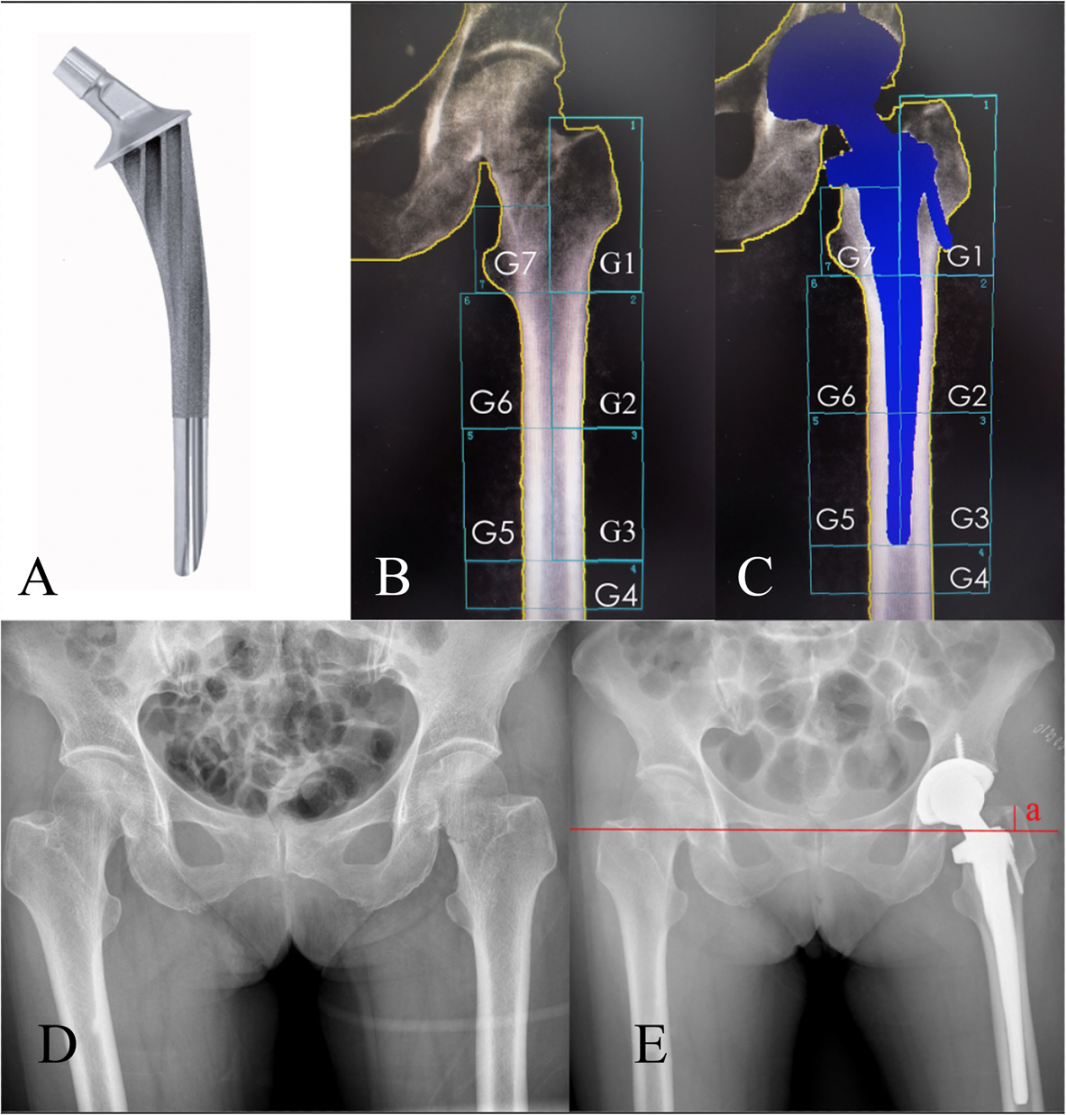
Seven Gruen zones used in Dual-energy X-ray absorptiometrical analysis of preoperative (a) and postoperative (b) periprosthetic BMD. Preoperative and postoperative anteroposterior X-rays of the pelvic were shown in (c and d). On anteroposterior X-ray of the pelvis, the inferior displacement of the femoral stem was evaluated by measuring the changes of the distance between the tip of greater trochanter and interteardrop line (Line a in d) during the follow-up period (a) showsed the appearance of the Ribbed anatomic stem (Waldemar Link GmbH & Co). Seven Gruen zones were used in Dual-energy X-ray absorptiometry analysis of preoperative (b) and postoperative (c) periprosthetic BMD. Preoperative and postoperative anteroposterior X-rays of the pelvic were shown in (d and e). On anteroposterior X-ray of the pelvis, the inferior displacement of the femoral stem was evaluated by measuring the changes of the distance between the tip of greater trochanter and interteardrop line (Line a in e) during the follow-up period
